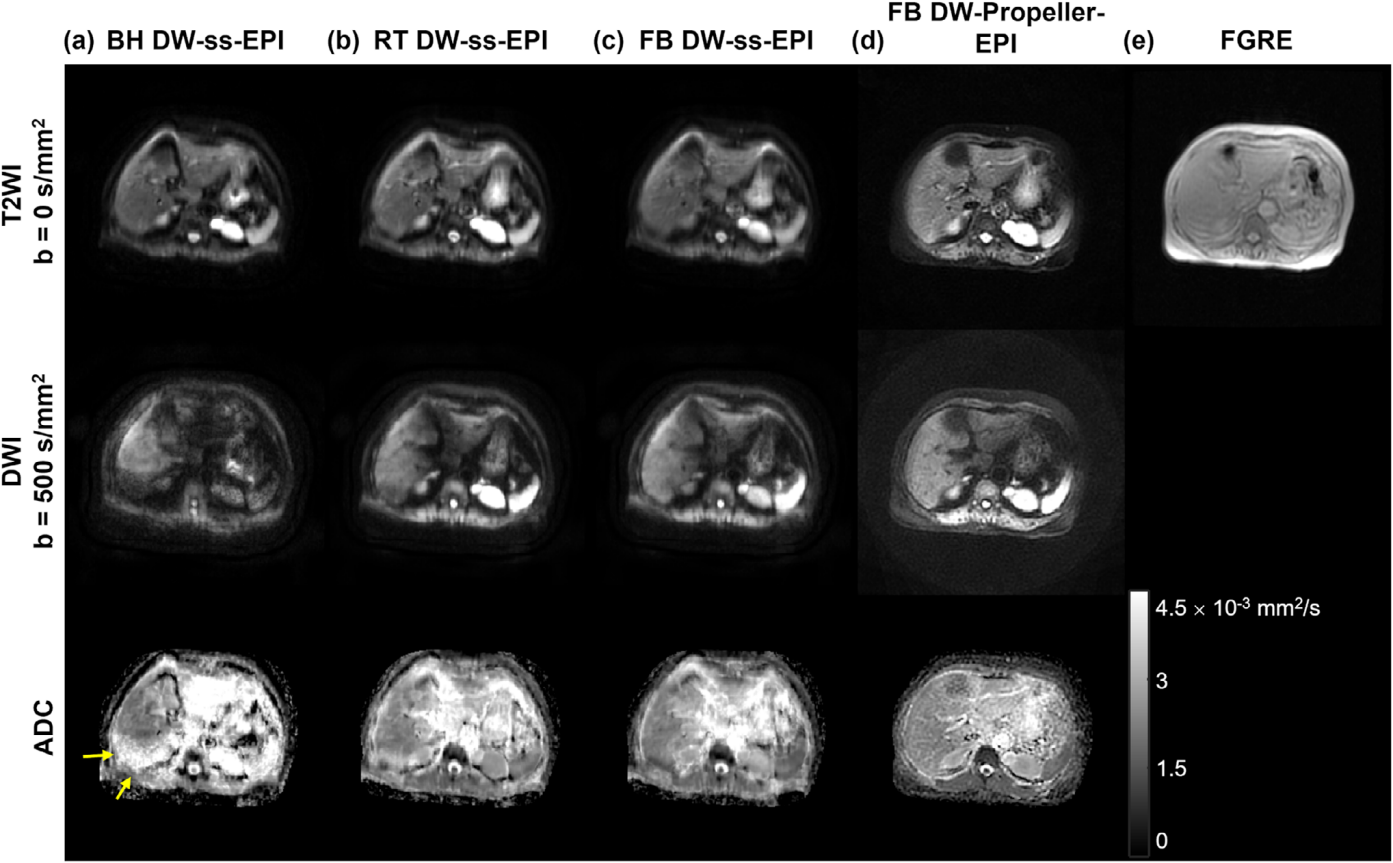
Representative liver diffusion images (at b‐value = 0 and 500 s/mm^2^) and corresponding ADC maps obtained from a 67‐year‐old woman with suboptimal breath holding, acquired using four techniques: (a) breath‐holding diffusion‐weighted single‐shot echo‐planar imaging (BH DW‐ss‐EPI), (b) respiratory‐triggering diffusion‐weighted single‐shot echo‐planar imaging (RT DW‐ss‐EPI), (c) free‐breathing diffusion‐weighted single‐shot echo‐planar imaging (FB DW‐ss‐EPI), and (d) free‐breathing diffusion‐weighted Propeller echo‐planar imaging (FB DW‐Propeller‐EPI). (e) Fast gradient‐echo (FGRE) images, serving as the gold standard for geometric fidelity. Yellow arrows indicate the prominent ADC calculation bias due to motion artifacts in the DWI image from the BH DW‐ss‐EPI technique. Based on evaluation by two radiologists, FB DW‐Propeller‐EPI yielded higher image quality scores than all three routine DW‐ss‐EPI techniques.
